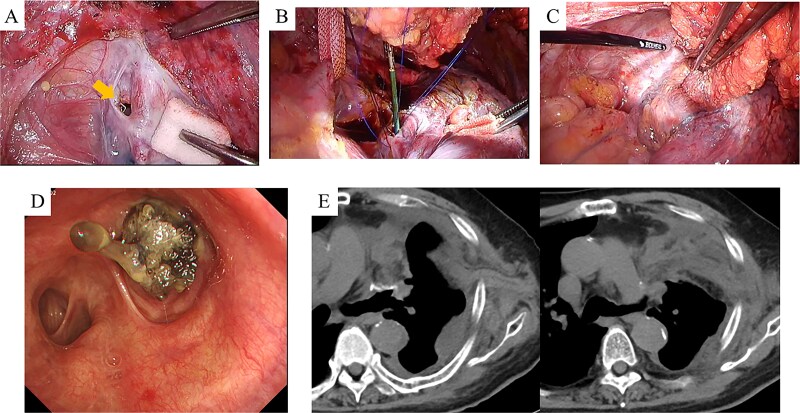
Intraoperative and early postoperative findings. (A–C) Panel A identifies the fistula at the upper lobe bronchial stump (arrow), adjacent to the pulmonary artery. Panel B shows a bronchoscope being used to guide the biopsy forceps from the fistula site into the thoracic cavity to grasp the latissimus dorsi muscle flap. Panel C shows the flap secured into the bronchial lumen in a retrograde fashion, with fibrin glue applied for reinforcement. (D) Bronchoscopic view on postoperative day 7 confirms visibility of the latissimus dorsi muscle flap within the bronchial lumen. (E) CT scans on postoperative day 7 demonstrate secure suturing of the latissimus dorsi muscle flap to the fistula site and resolution of the preoperative airspace.
